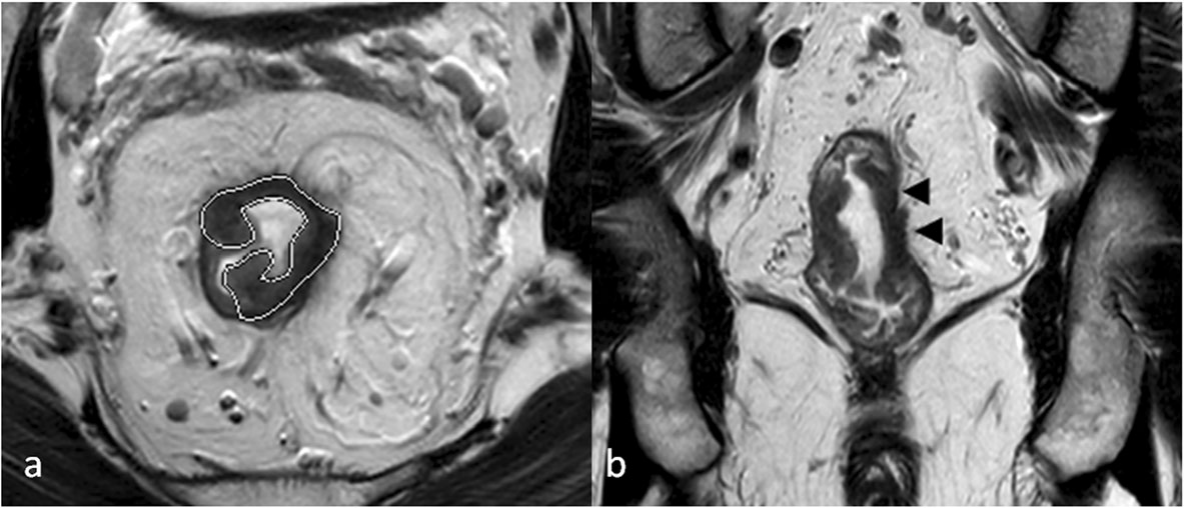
T2-weighted imaging of a rectal cancer (low to intermediate signal intensity) presenting as ulceroinfiltrative mass (a, oblique axial, outline indicates tumor region) mainly extended along the intestinal wall with ambiguous muscularis propria (b, coronal, arrow head). Final pathologic results demonstrated that tumor invaded through muscularis propria to perirectal tissues
